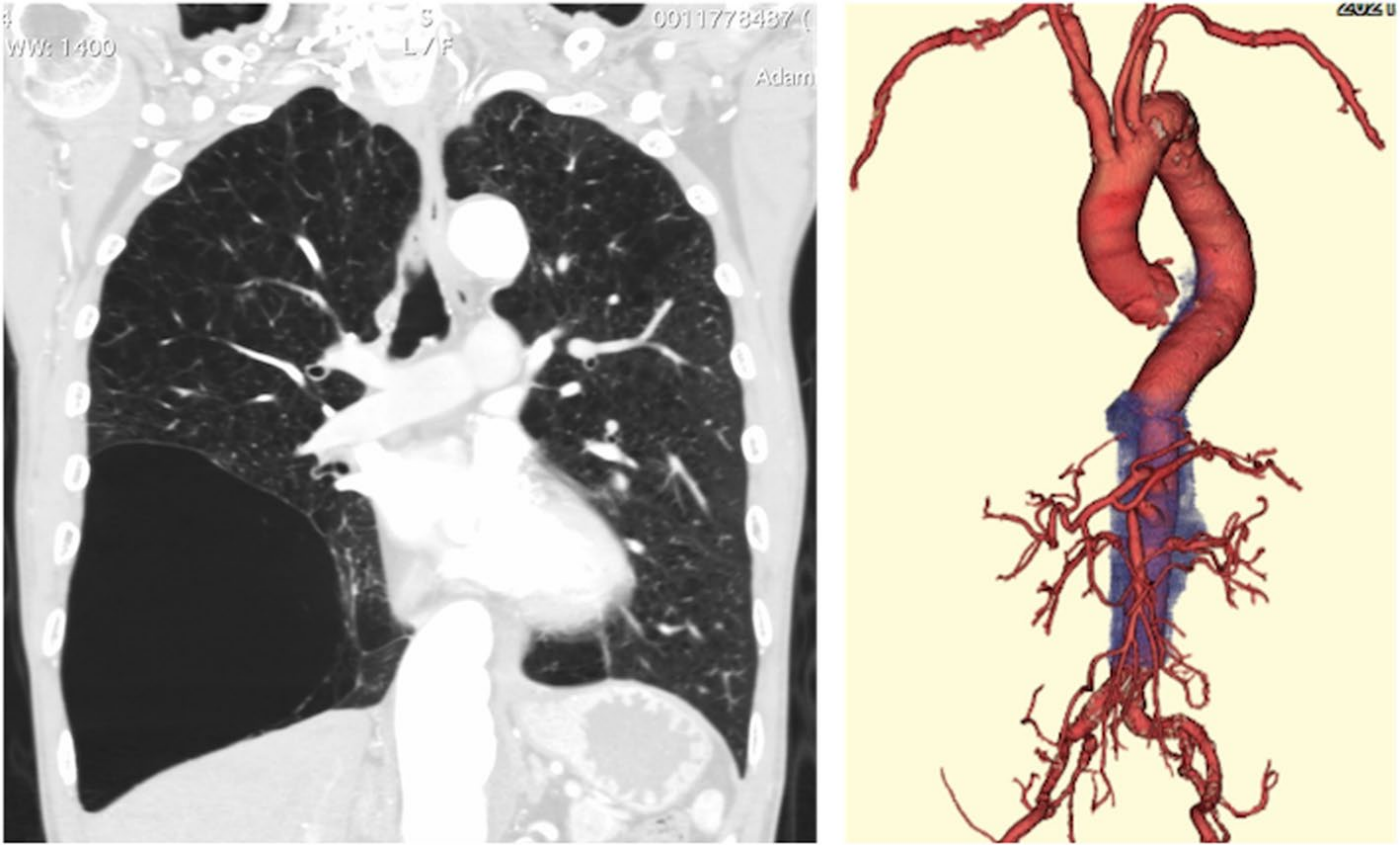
The patient’s preoperative computed tomographic findings. Left: a giant bulla in the right lung. Right: three-dimensional image of the thoracoabdominal aneurysm
